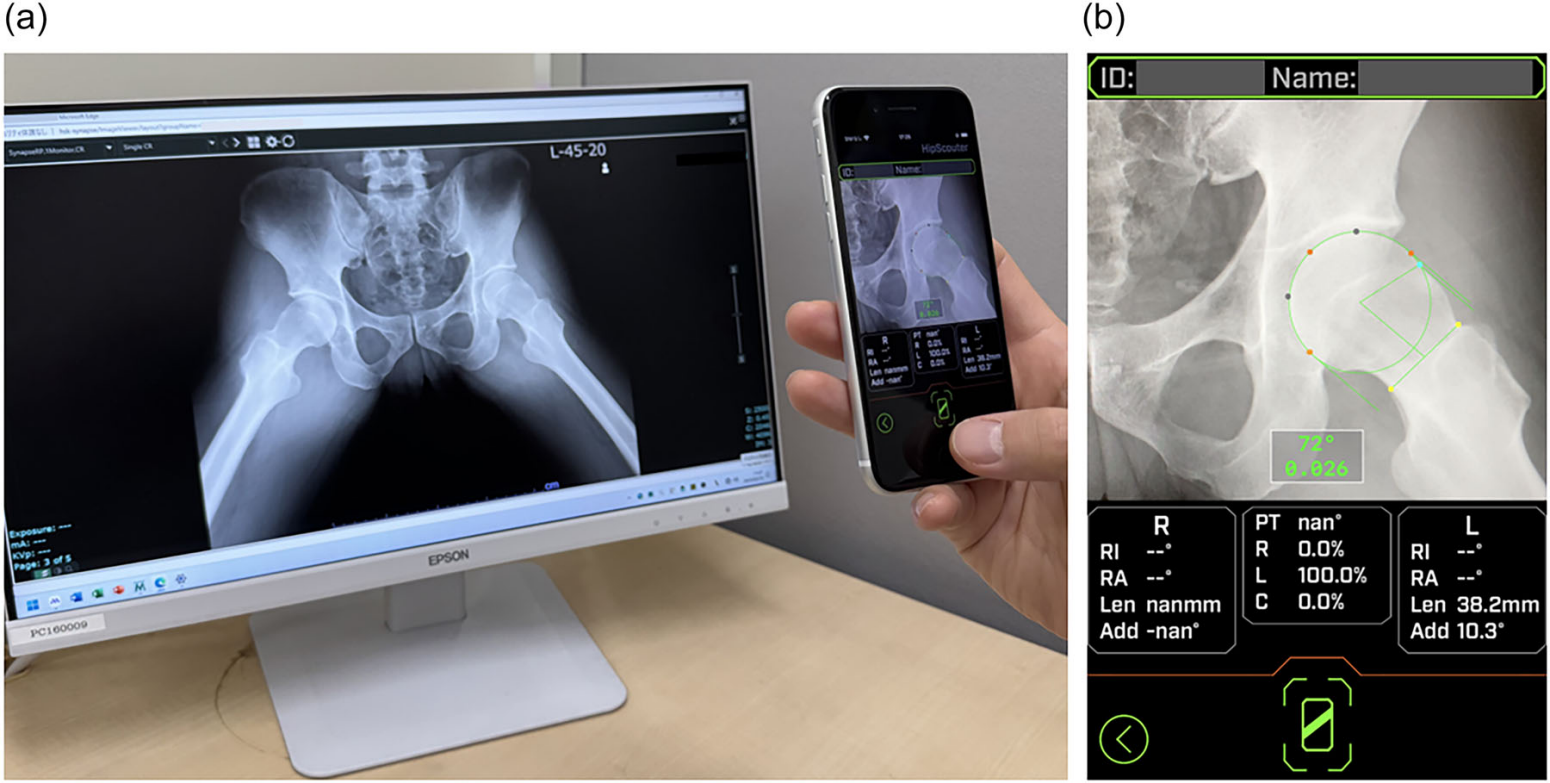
Artificial intelligence‐powered system for the immediate measurement of alpha angle. (a) Alpha angle is measured by capturing a 45° Dunn view radiograph using a smartphone camera. (b) Screenshot image of (a).
